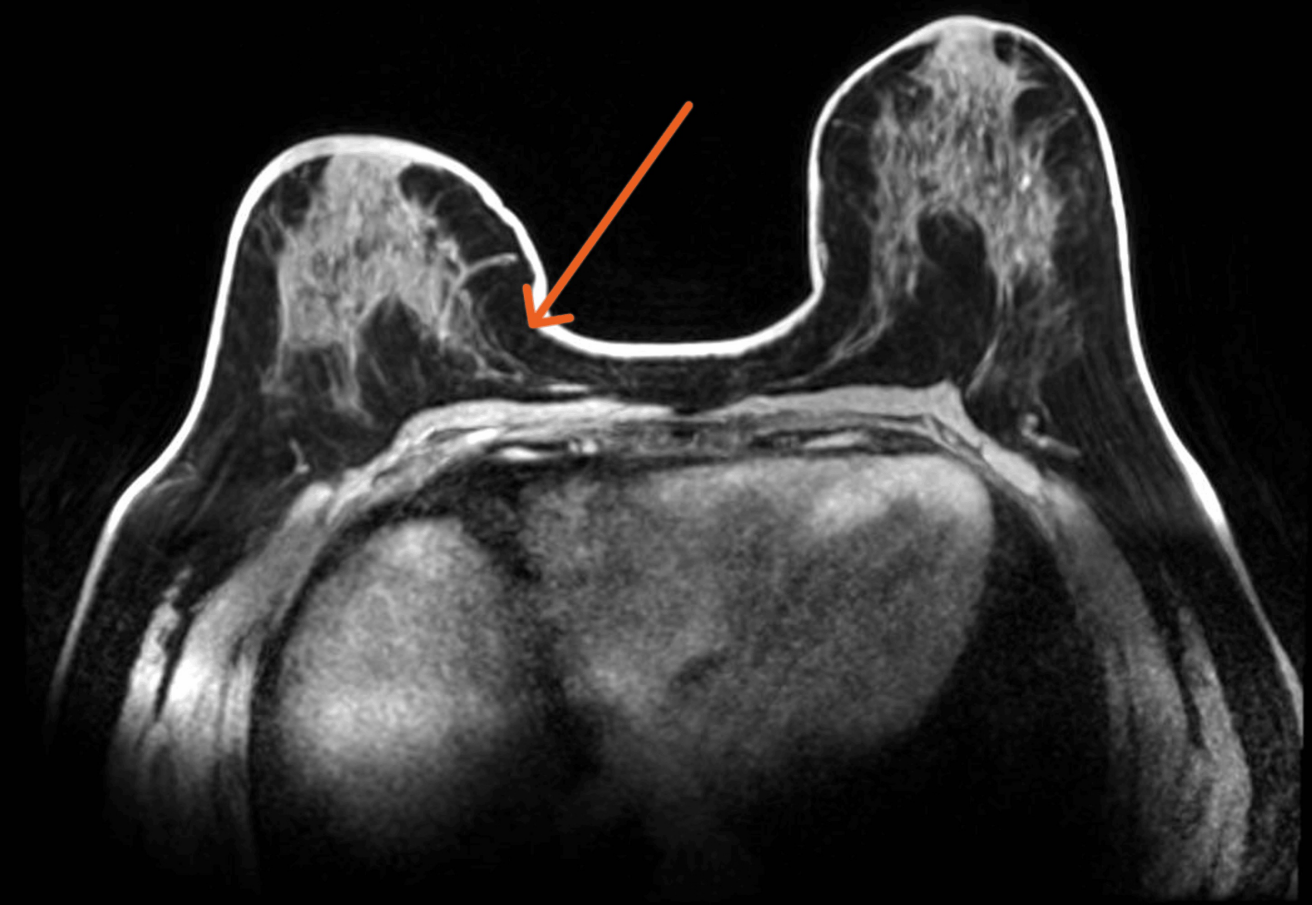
Post-chemotheraphy right breast MRI showing no areas of suspicious enhancement in the area where the primary tumor was previously noted (orange arrow).
